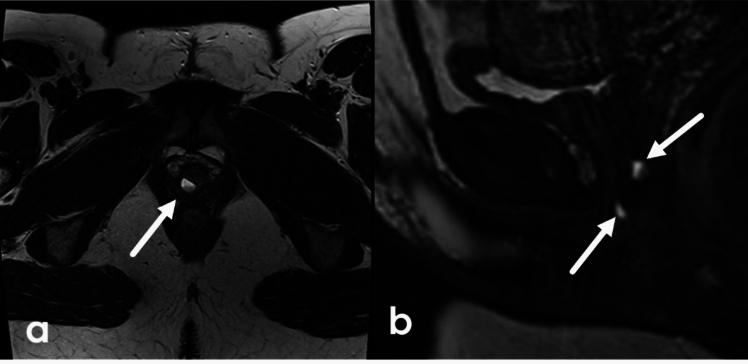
37-Year-old female undergoing pelvic MRI for abnormal uterine bleeding with incidentally noted periurethral cyst. Skene’s gland cysts are often located lateral to the urethra. This may help in distinguishing them from urethral cysts which may be more posterior in location
